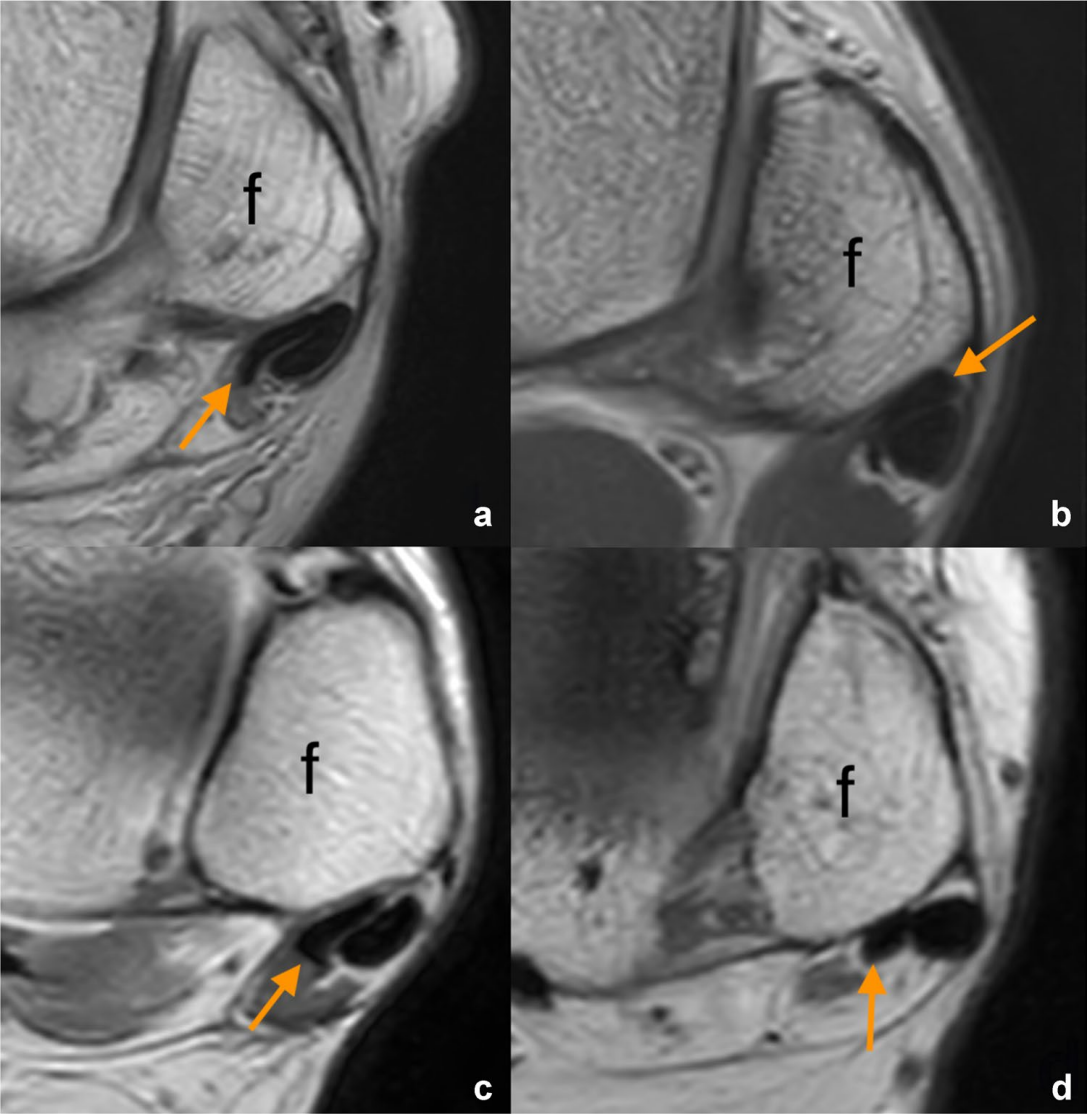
Axial section at the level of the lateral malleolus and the ankle joint space (magnetic resonance images with proton-density weighting, left sides). The four morphological types of the peroneus brevis tendon (arrow) are as follows: a general flat, b flat with a lateral bulge, c flat with a medial bulge, and d oval tendon. The fibula is labeled with f
